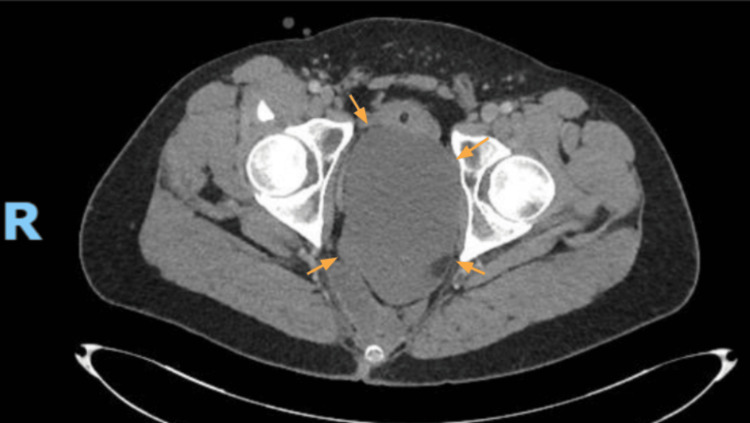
Abdominopelvic axial CT A large, lobulated pelvic mass is identified, measuring 159 × 95 × 88 mm. The lesion demonstrates low attenuation, slightly above that of simple fluid. It exerts a significant mass effect, resulting in anterior and superior displacement of the seminal vesicles and urinary bladder, as well as contouring and rightward displacement of the sigmoid and rectosigmoid colon.
